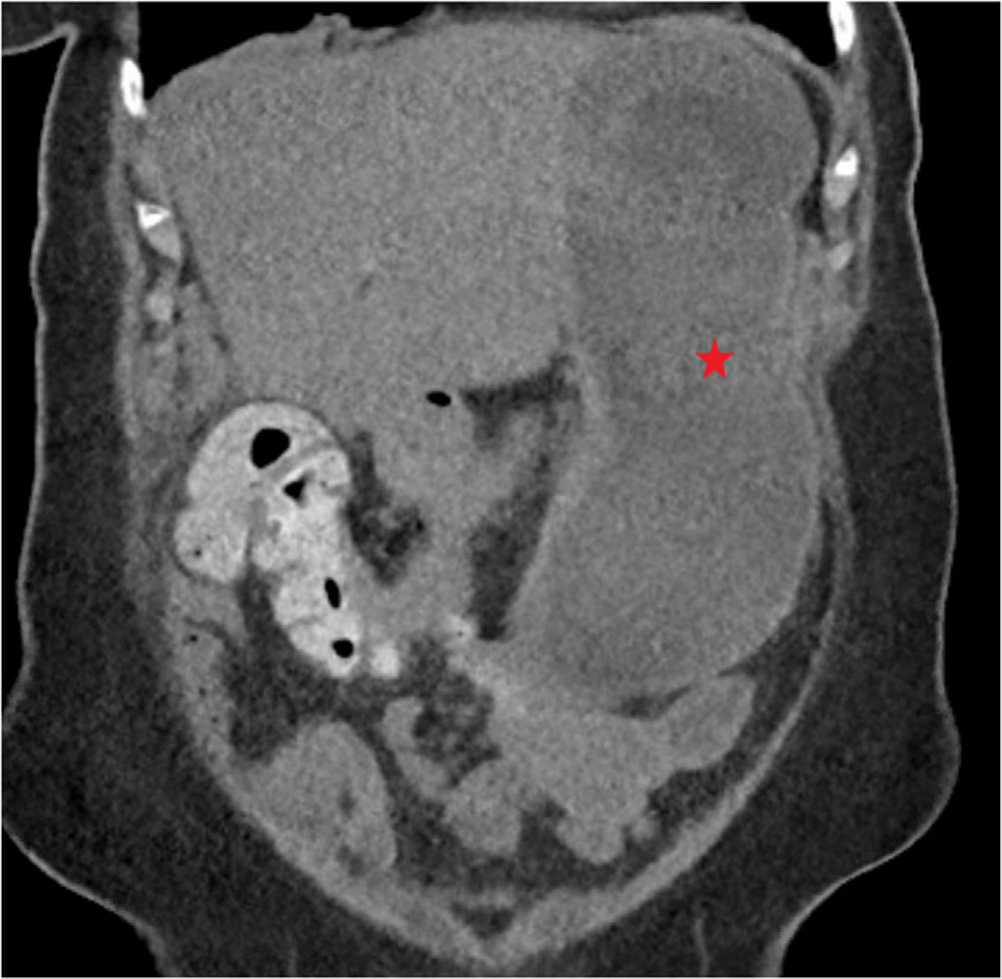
Computed tomography scan showing gastric distension (red star) with fluid and gas attributable to gastric outlet obstruction from a duodenal adenocarcinoma.
